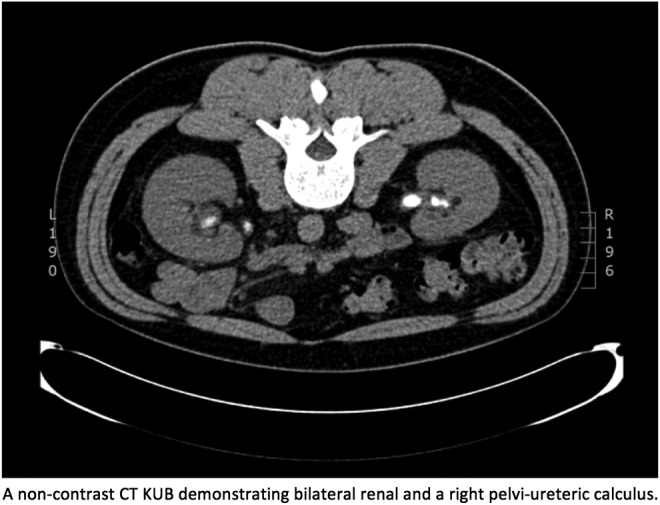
A noncontrast CT KUB showing bilateral renal and a right pelviureteral calculus. KUB, kidney, ureter, and bladder radiograph.
